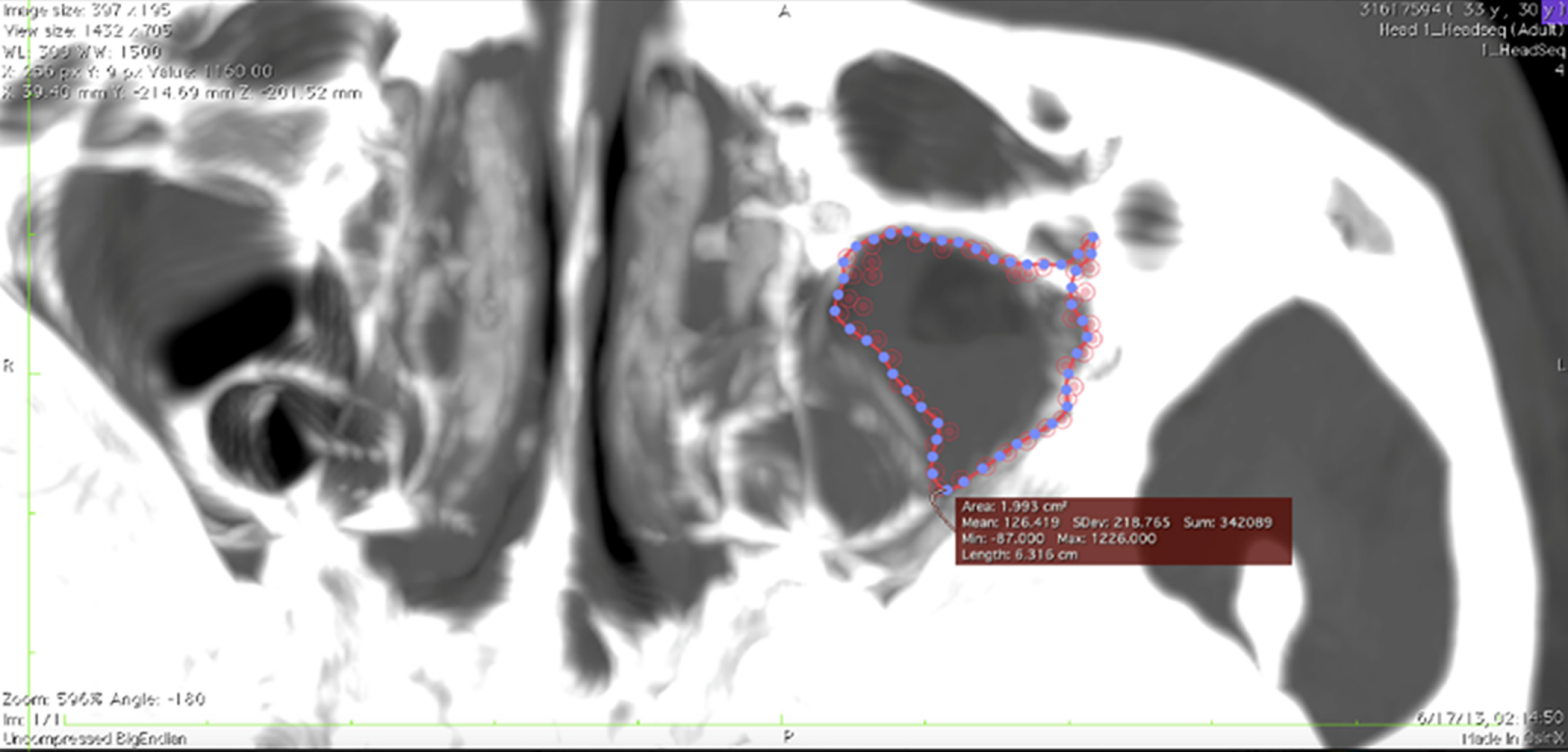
The axial computed tomography with area of fracture. The area of fracture is automatically calculated using the pencil tool in the OsiriX v5.8.2 software.
